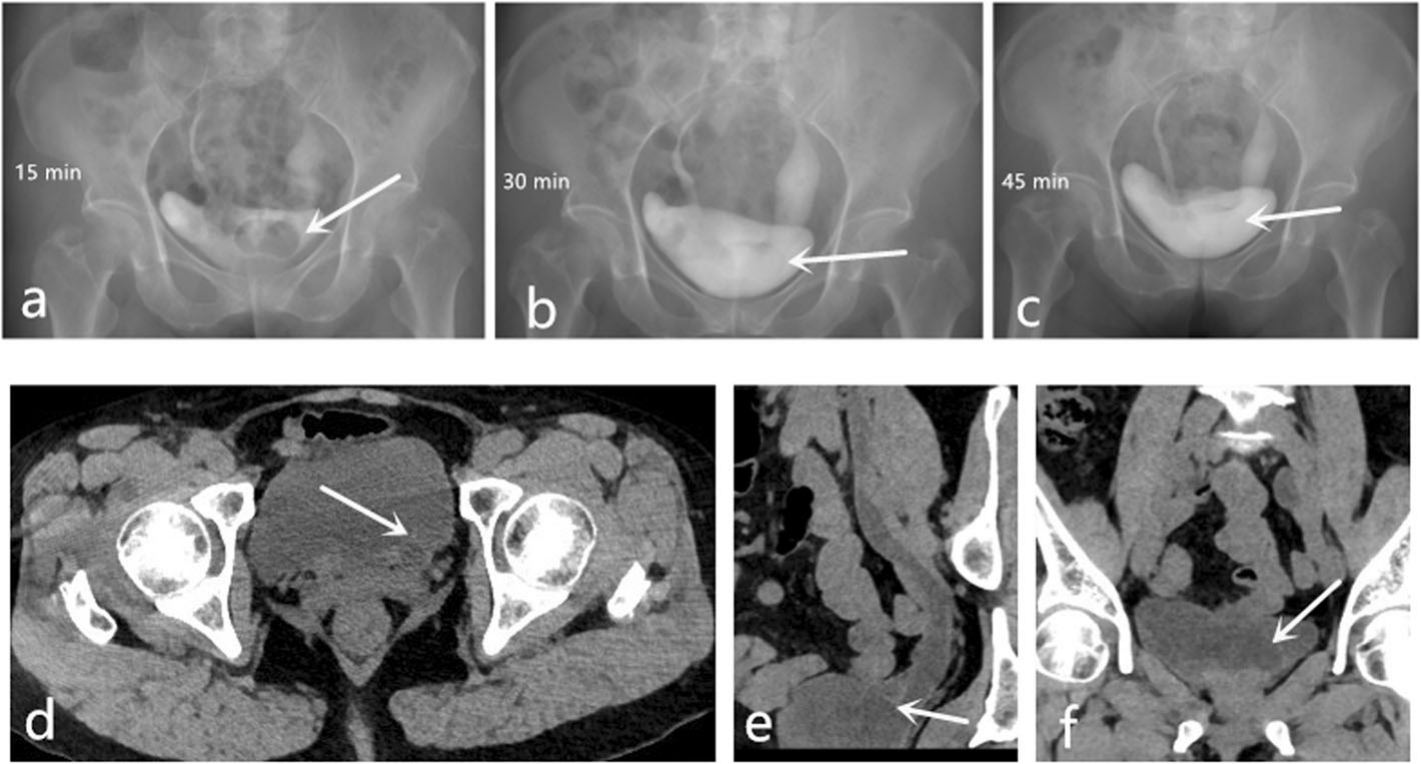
Intravenous pyelogram at 15 (a), 30 (b), 45 (c) minutes respectively after injecting contrast medium revealed a ureterocele. CT scan (d, e, f) revealed that a ureterocele was located in the left ureterovesical junction
